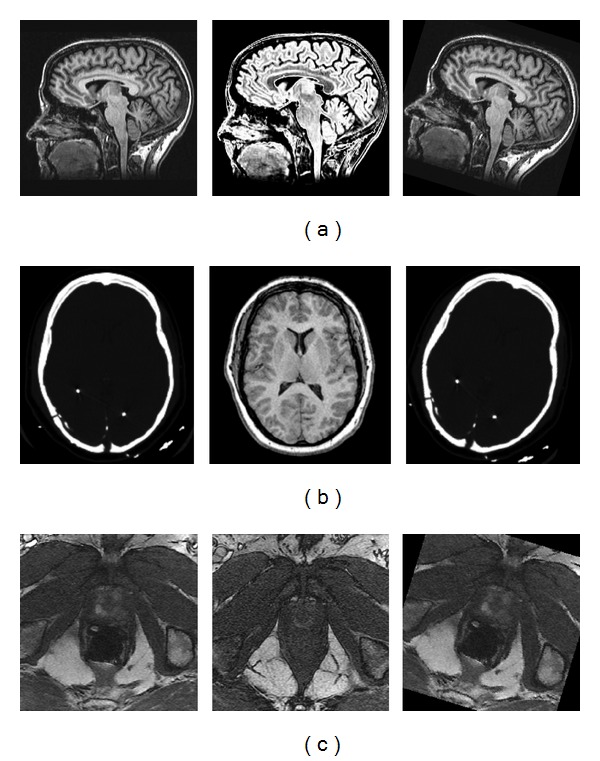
Image pairs used to evaluate the registration method. The first column and the second column were the original image pairs and the third column was generated by rotating the first column image with fixed 17° clockwise. (a) Brain T1/T2 MRI with intensity and slight scale difference. (b) Head CT/MR with significant differences. (c) Prostate MR with deformations and partial occlusions due to the surgery.
